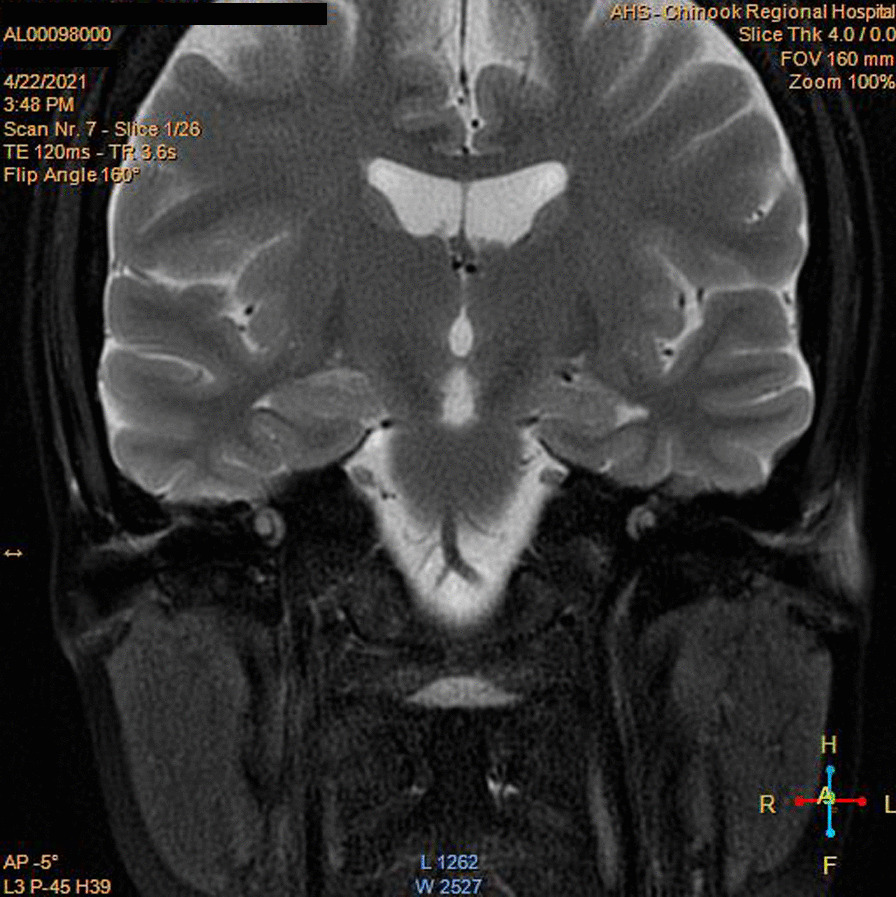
Orbit and cervical MRI of patient during pembrolizumab therapy. Magnetic resonance imaging (MRI) show symmetric appearance of the larynx, vocal cords, and hypopharynx soft tissues. Submandibular glands have a fairly symmetric appearance. Previously seen abnormality on the right is not present today. There is no evidence of identified adenopathy or abnormal soft-tissue enhancement
